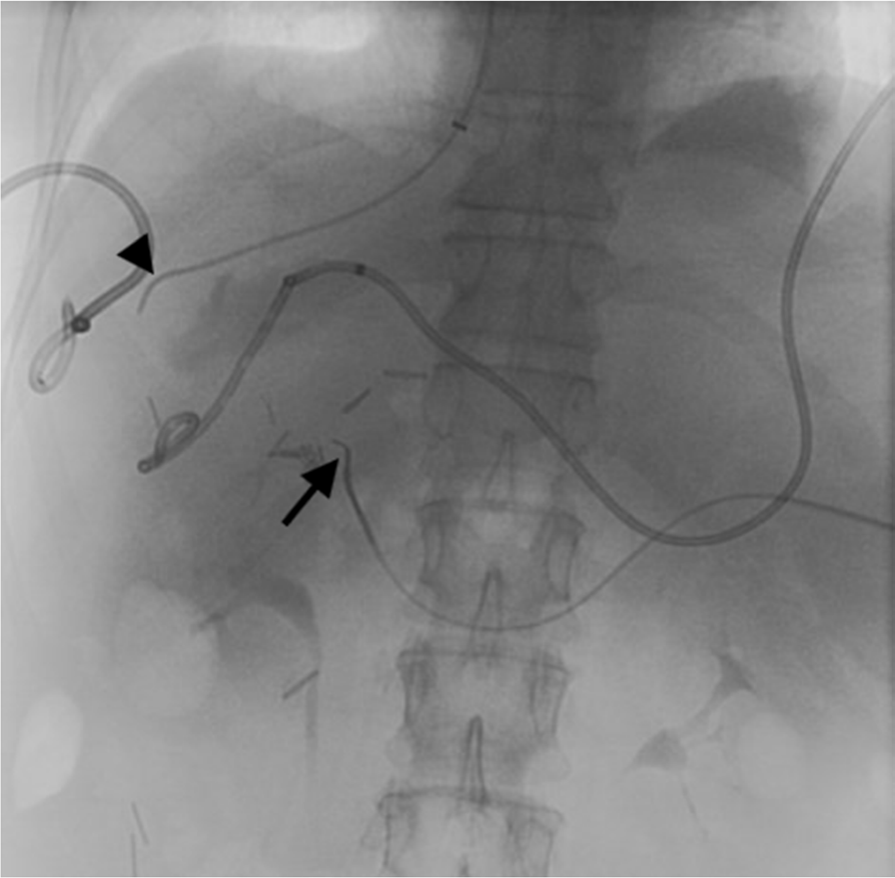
CBCT demonstrates the spatial relationship between the trans-splenic catheter situated in the region proximal to the ligated portal vein (black arrow) and the catheter in the middle hepatic vein (black arrowhead)
